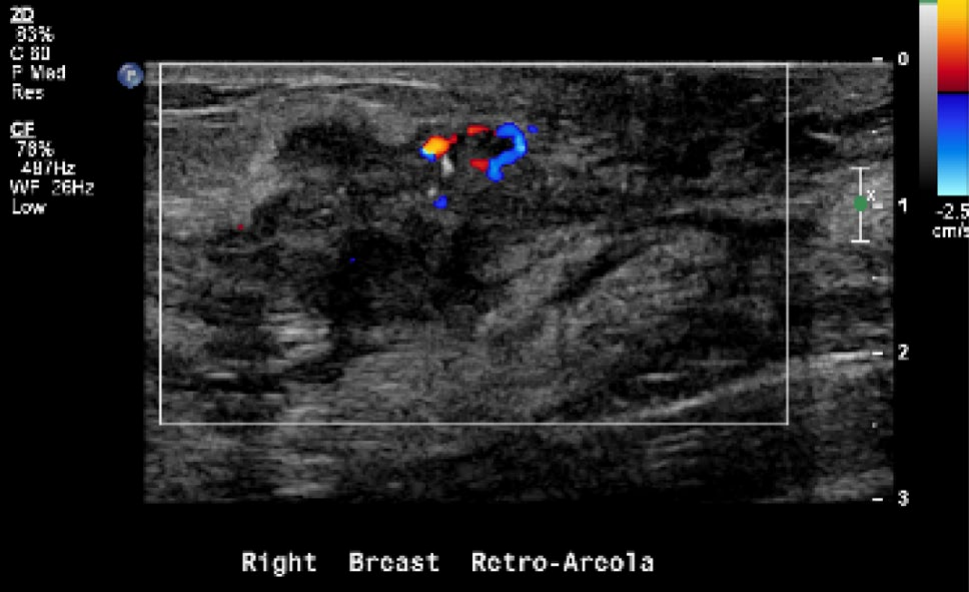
The ultrasound for the same patient shows an irregular-shaped mass with calcifications at retroareolar region
